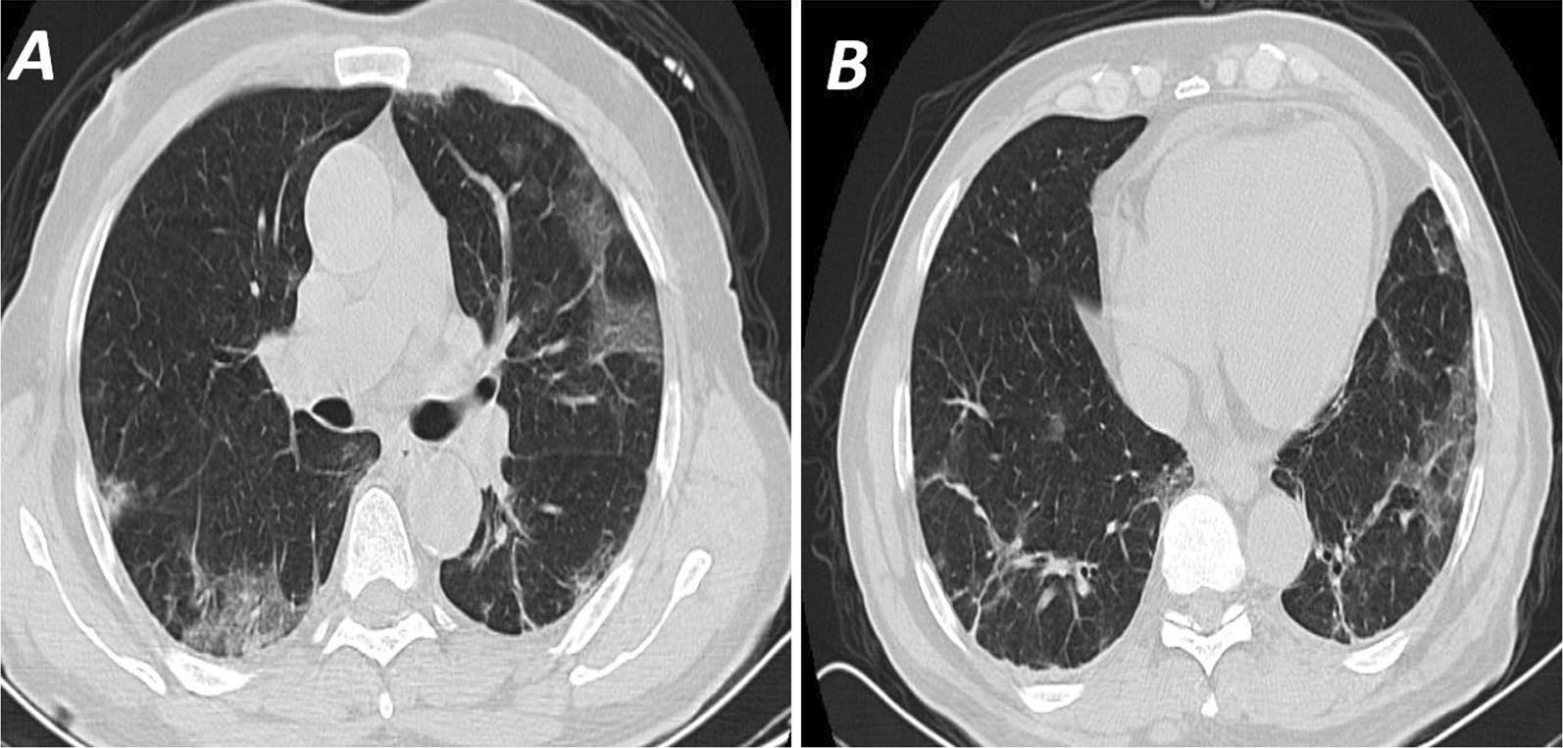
A and B High-resolution CT scan (axial cuts) for a 60 year-old diabetic male patient presented with fever and cough. CT shows multiple bilateral peripheral patches of ground-glass opacities in both lung lobes with linear consolidations seen in both lower lung lobes interpreted by all readers as a high probability (CO-RADS 5). RT-PCR was positive for COVID-19 and the patient responded to treatment and was discharged 14 days later
